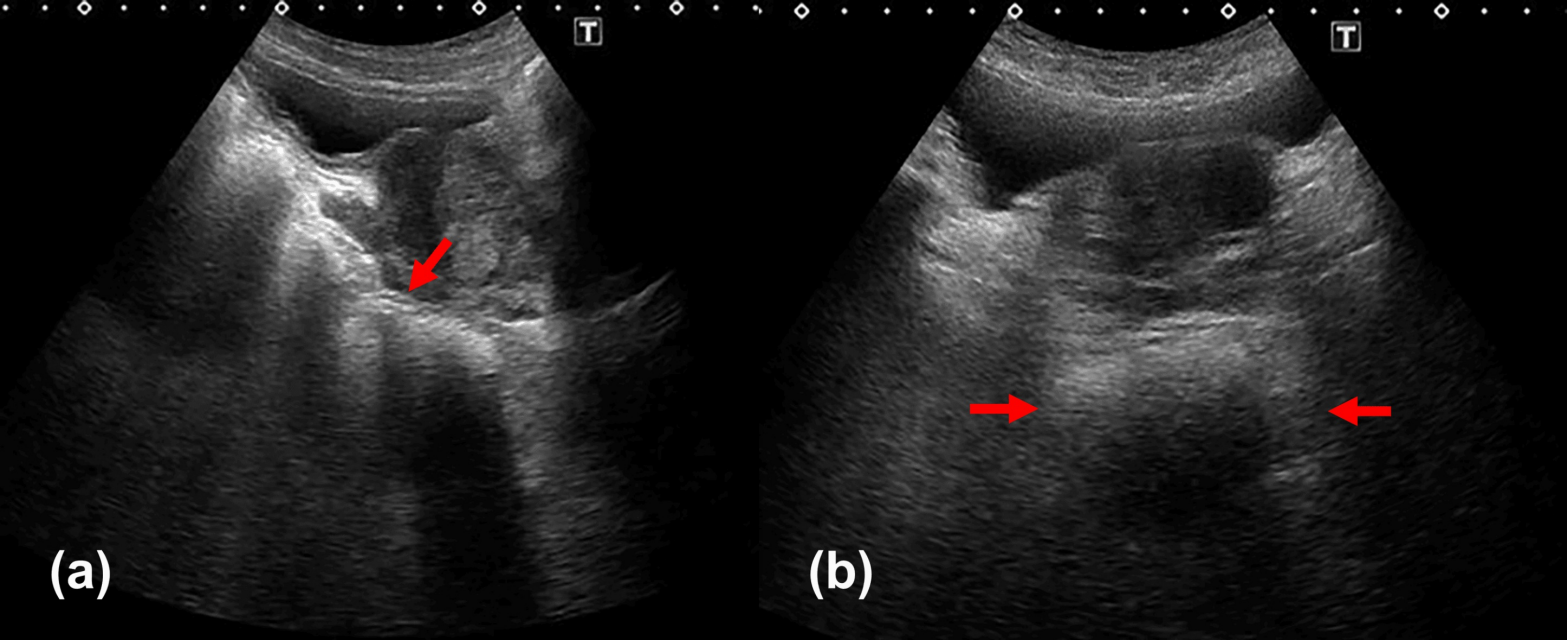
Short-axis and long-axis ultrasonographic images of the rectum on day 1 (a) A hyperechoic mass with posterior acoustic shadowing is noted in the rectum, suggestive of a fecal impaction. The rectum and proximal colon are dilated and filled with hyperechoic material, consistent with constipation. (b)The transverse diameter of the rectal distension on the short-axis view measures approximately 46 mm. Rectal diameter was measured from outer-wall to outer-wall in the transverse plane, following the method described by Yabunaka et al. [11]. The patient was examined in the supine position. Images were obtained using an Aplio 500 ultrasound system (Canon Medical Systems Corporation, Otawara, Japan) with a convex probe set at 3.5 MHz
